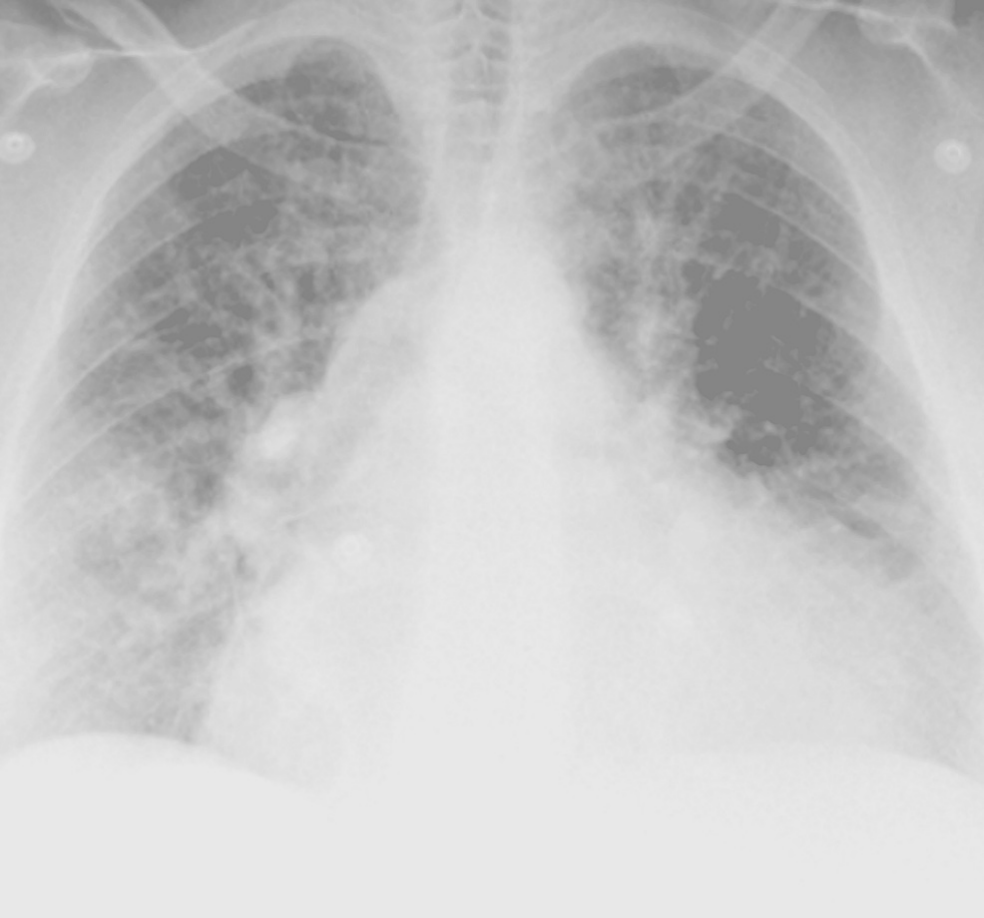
Chest X-ray showing widespread interstitial infiltrates and cardiomegaly due to pulmonary edema.
